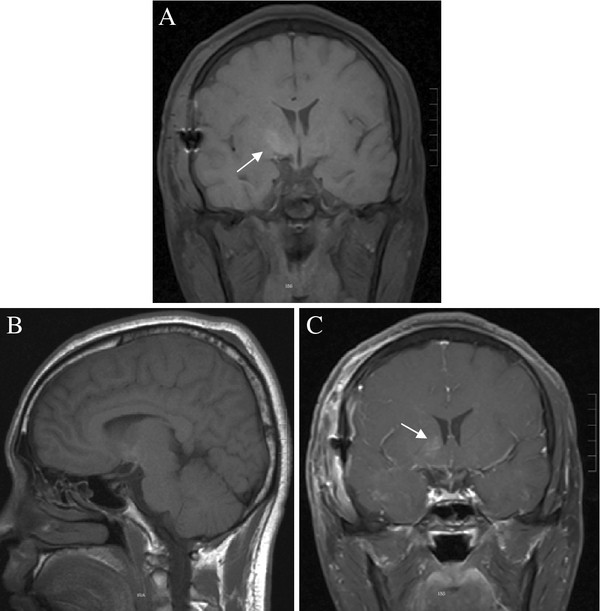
Magnetic resonance images of postoperative follow-up. (A,B) MRI scanning and (C) enhanced scanning revealed the complete removal of chiasmal cavernous malformations and no recurrence, but a hyperintensity in the basal ganglia was found (arrows).
